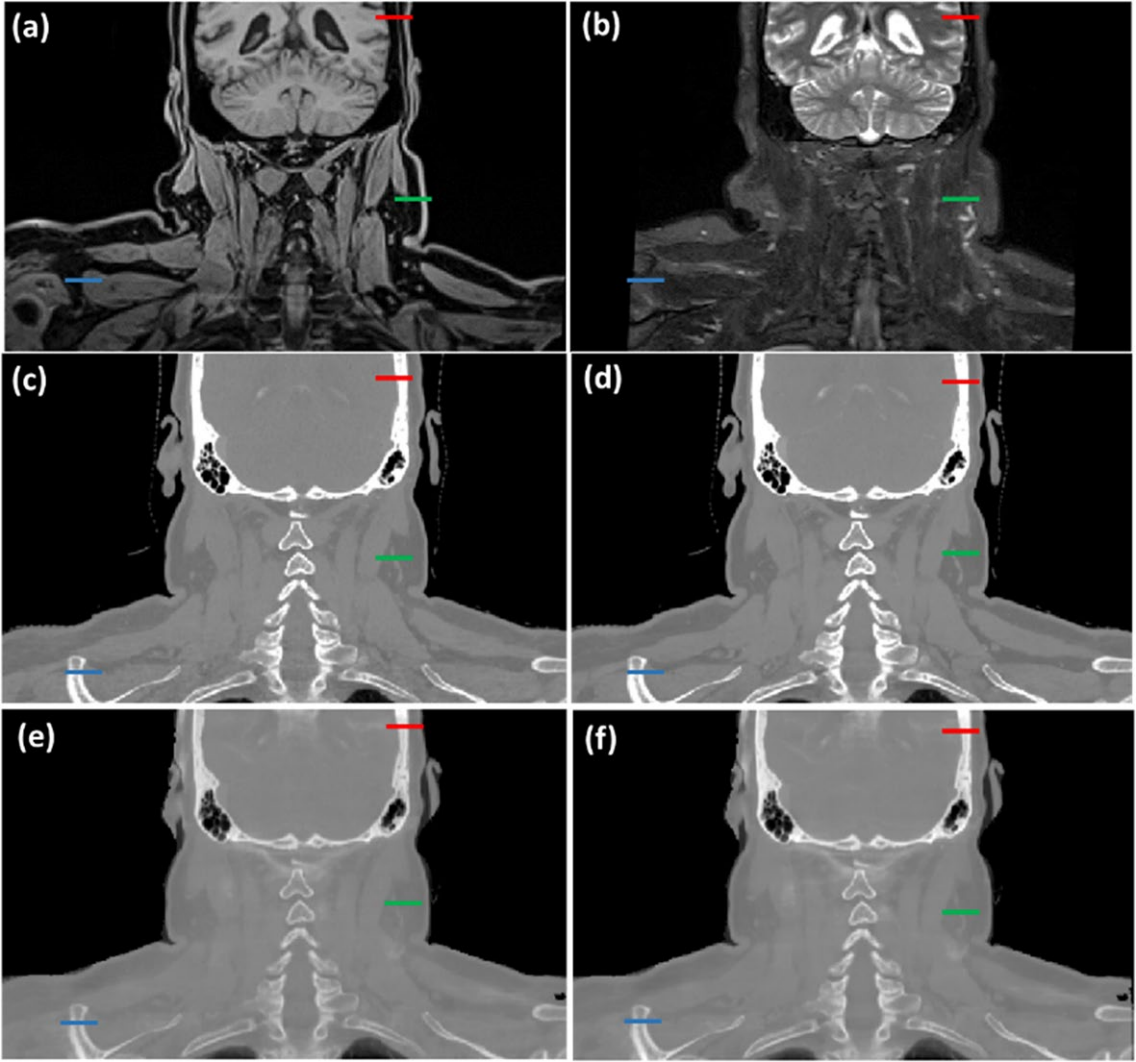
Patient coronal images from, (a) T1-weighted Dixon predicated water-only (T1-D-P-W), (b) T2-weighted short tau inversion recovery (T2-stir), (c) DECT high-energy, (d) DECT low-energy, (e) sDECT high-energy, (f) sDECT low-energy. (a,b) are MR scans image from the patients, (c,d) are the DECT scans for that patient, (e,f) are the synthetic DECT images generated based on (a,b) using established method describe in our previous work^37^. The red, yellow, and green bars indicate specific locations used for line profile comparison, as demonstrated in the results presented in Fig. 6. The profile comparisons are shown in Fig. 7.
